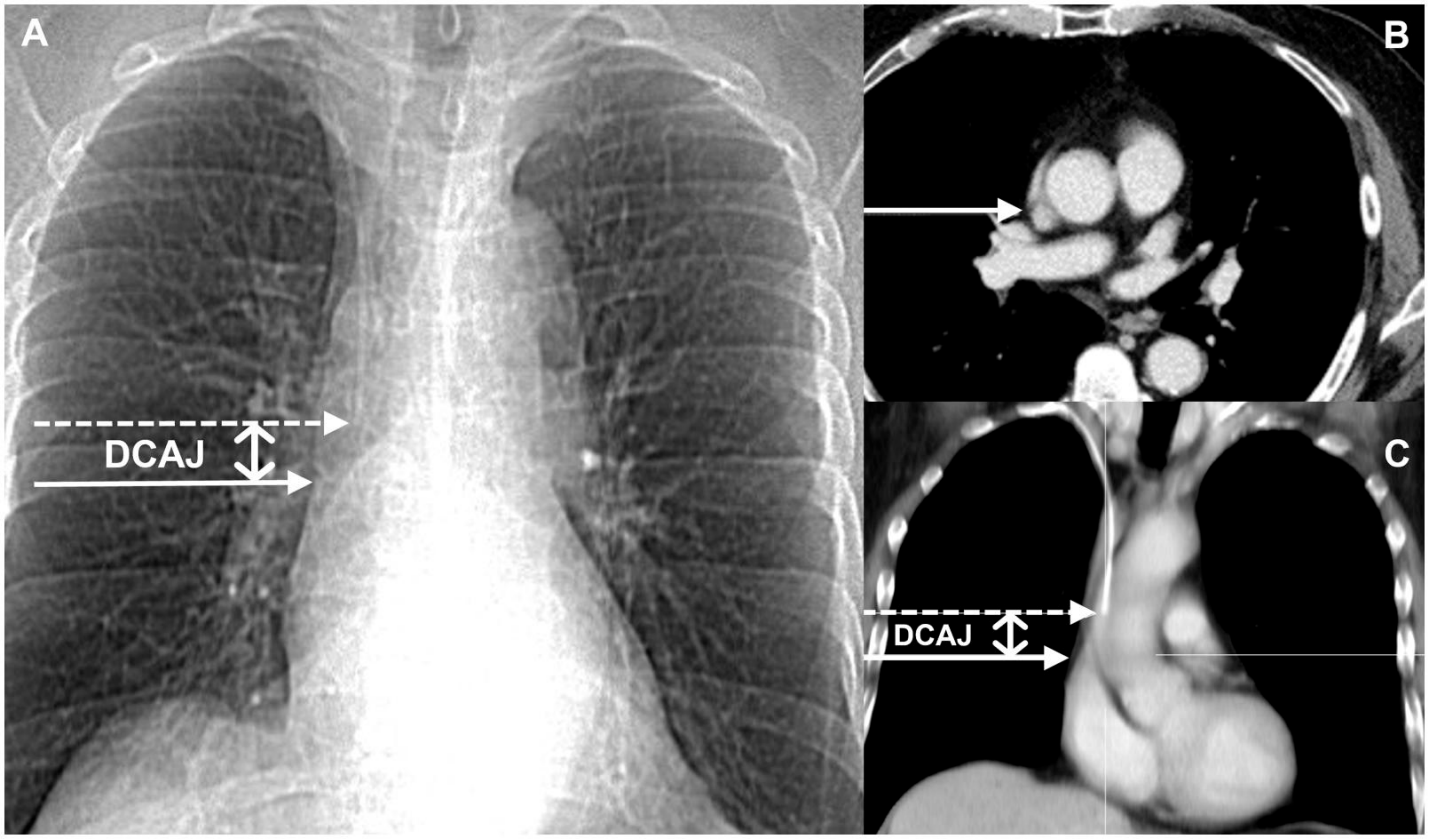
(A) Projectional CT image. Intersection between the right lateral margin of the superior vena cava (SVC) and the superior border of the right atrium determines the level of cavo atrial junction (CAJ) (closed arrow). The catheter tip is identified in the SVC (dashed arrow). (B) Axial CT image. Fat-filled (hypodense) band (closed arrow) determines the crista terminalis and thus the level of CAJ. (C) Multiplanar CT reconstruction. The plane of the axial image containing the CAJ is reported on the multiplanar reconstruction (closed arrow). The catheter tip is identified in the SVC (dashed arrow). (A + C) DCAJ is the vertical distance from the catheter tip to the CAJ (double open arrow).
